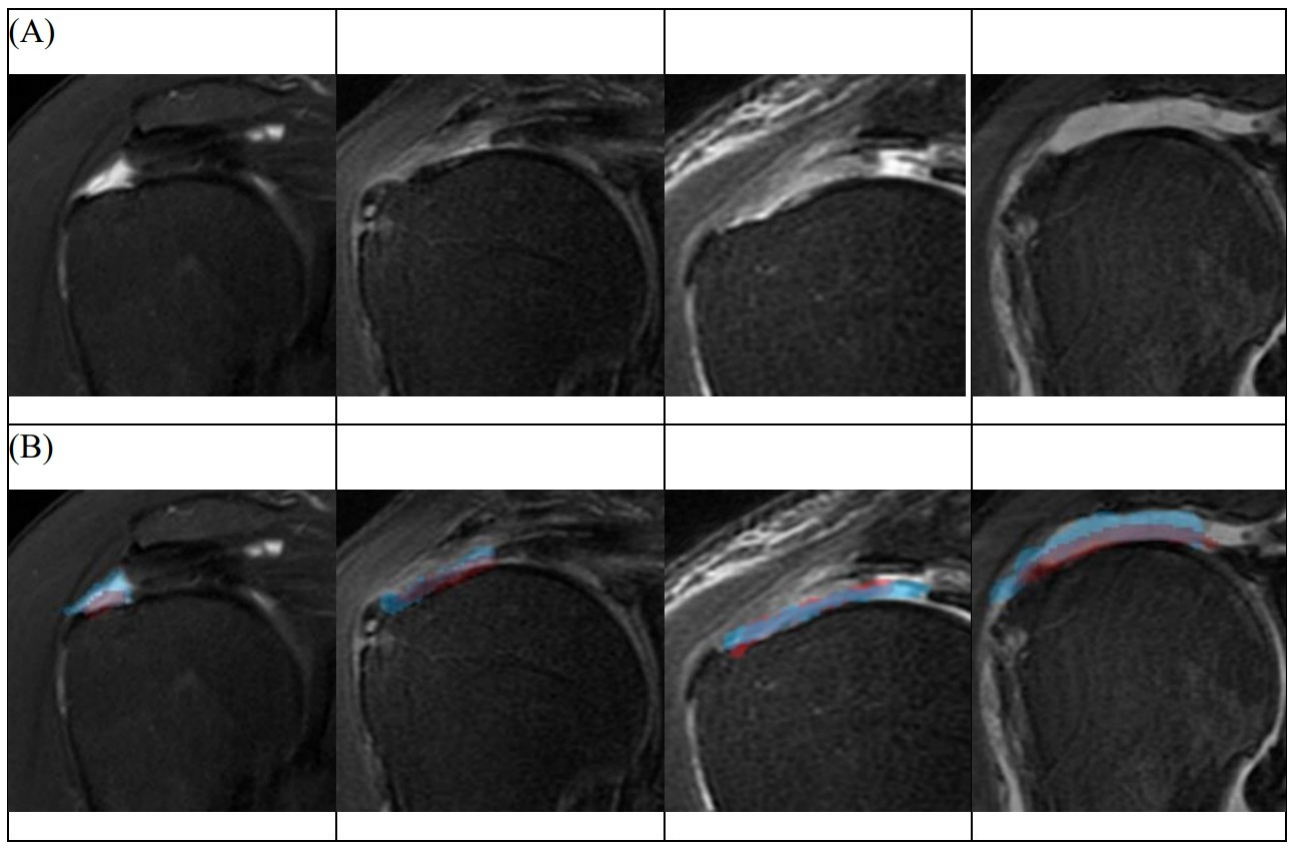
Results of segmentation corresponding to rotator cuff tear site. (A) Examples of the original MRI image which show rotator cuff tear. (B) The red area indicates the manually labeled region by shoulder specialists, and the blue area indicates the segmented region by the proposed learning model.
